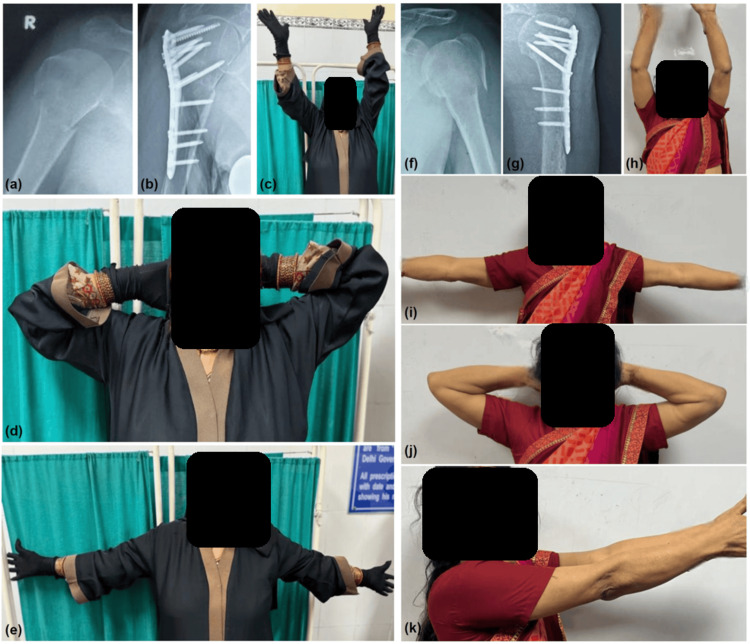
Preoperative X-ray, X-ray, and clinical photographs at one-year follow-up visit a-b) Preoperative X-ray and X-ray at 1-year follow-up, respectively, of patient no. 10 of the study. c-e) Clinical photographs taken at the 12-month follow-up visit demonstrating the functional outcome of patient no. 10. f-g) Preoperative X-ray and X-ray at 1-year follow-up, respectively, of patient no. 3 of the study. h-k) Clinical photographs taken at the 12-month follow-up visit demonstrating the functional outcome of patient no. 3.
